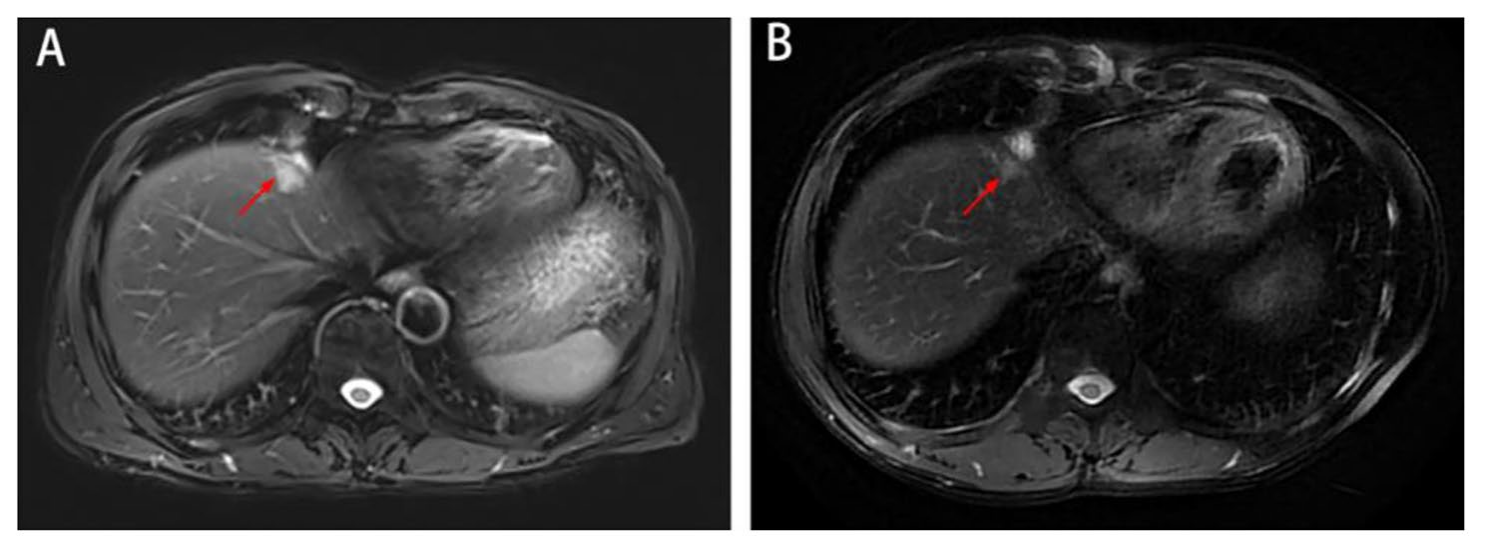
Liver metastatic lesions were significantly reduced after chemotherapy plus targeted therapy. A: In April 2022, MRI showed that the metastatic lesion measured 1.9 cm(red arrow). B: In June 2022, MRI showed a lesion (1.3 cm)in S4 of the liver (red arrow), and no new metastatic lesions were detected
